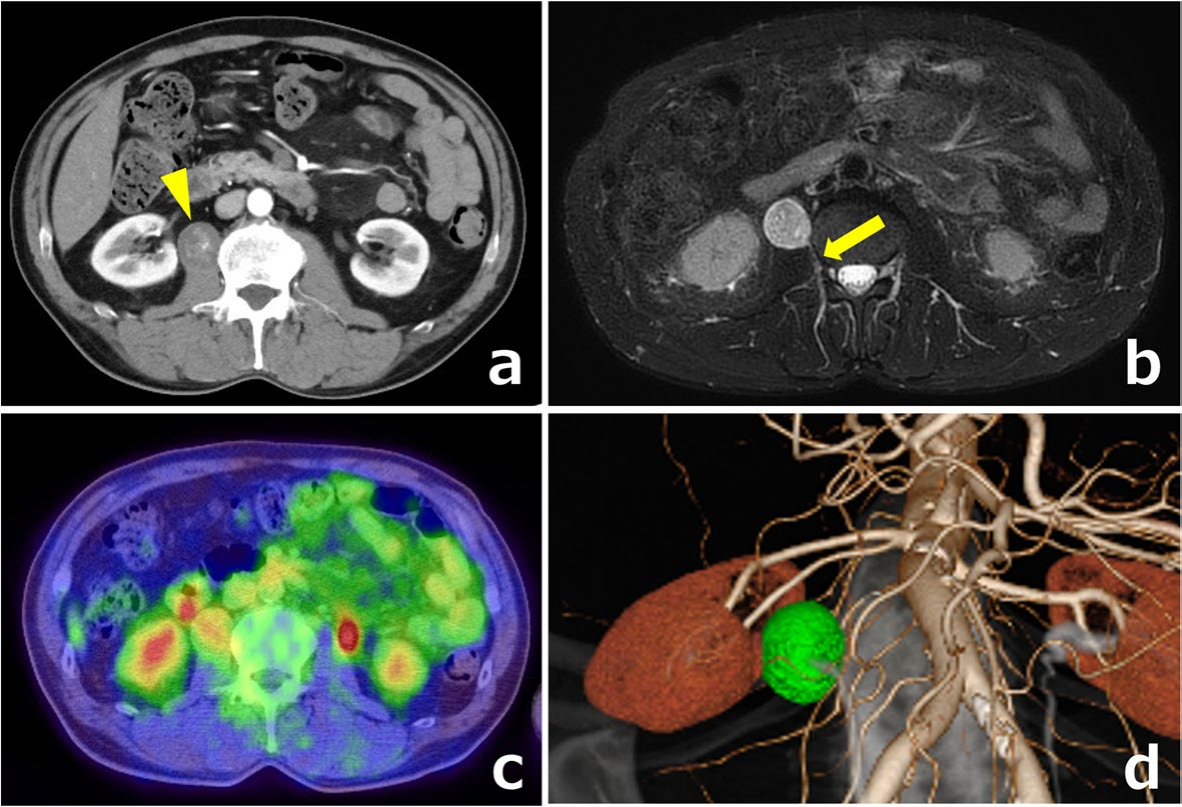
a CT showed a well-defined round 27 × 24 mm cystic mass (yellow arrowhead). b T2-weighted MRI showed a high-intensity lesion with the main feeder being a branch of the right third lumbar artery (yellow arrow). c PET-CT showed a mass with low FDG uptake. d The locations of the tumor and main feeder were identified before surgery on 3D images
